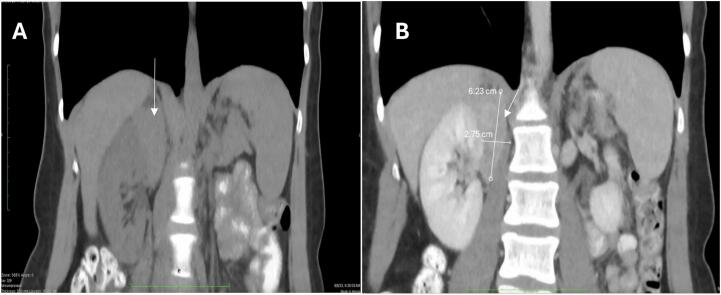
(A) and (B) Abdominal CT scan without and with contrast showing an enhancing mass measuring 6.2 × 2.7 × 5.5 cm. The mass is said to originate from the right kidney or from the tip of the medial limb of the adrenal gland.
